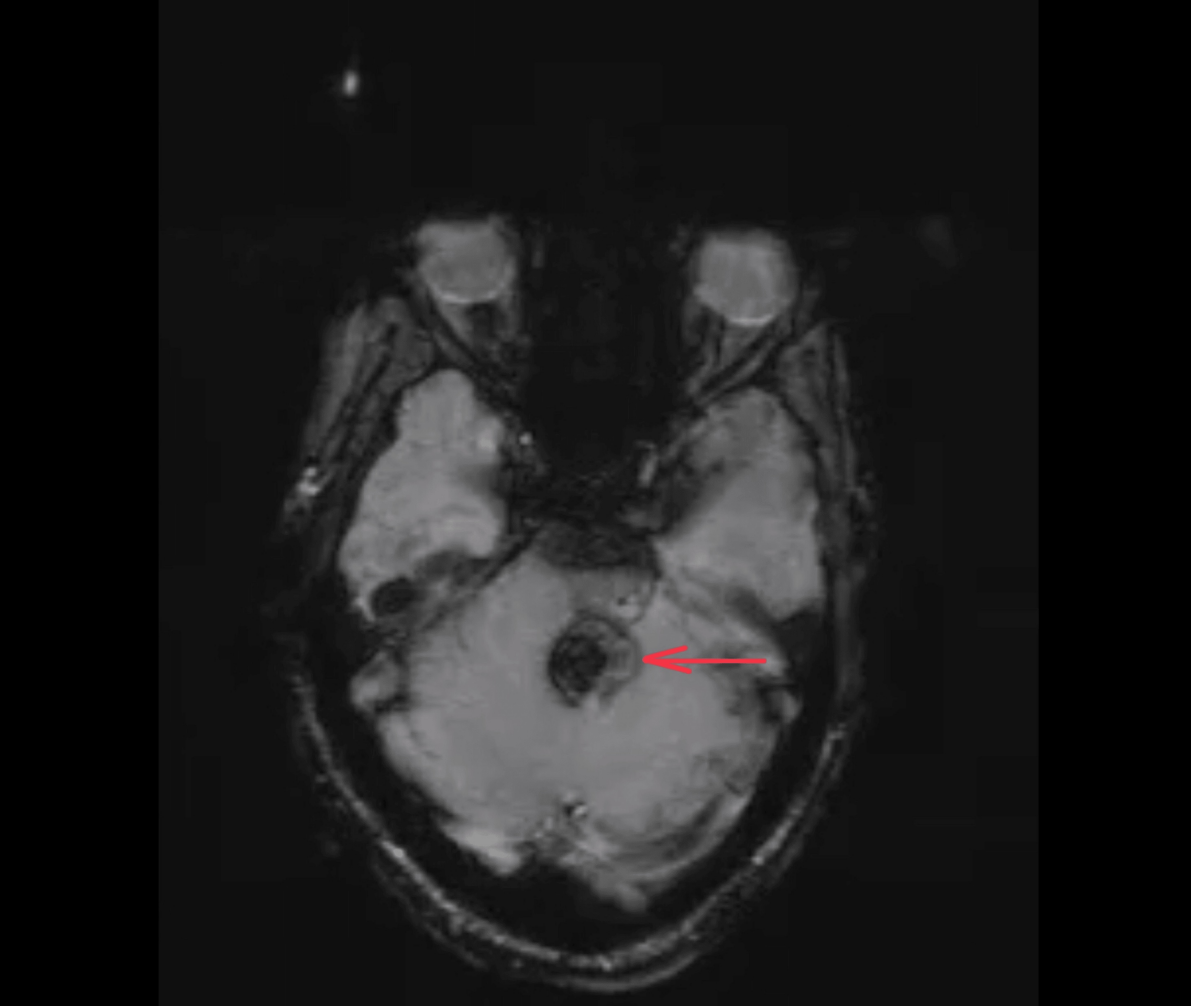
MRI brain: SWI showing areas of SWI blooming noted within likely to represent hemosiderin deposition pointed using red arrows SWI: Susceptibility weighted imaging; MRI: magnetic resonance imaging
